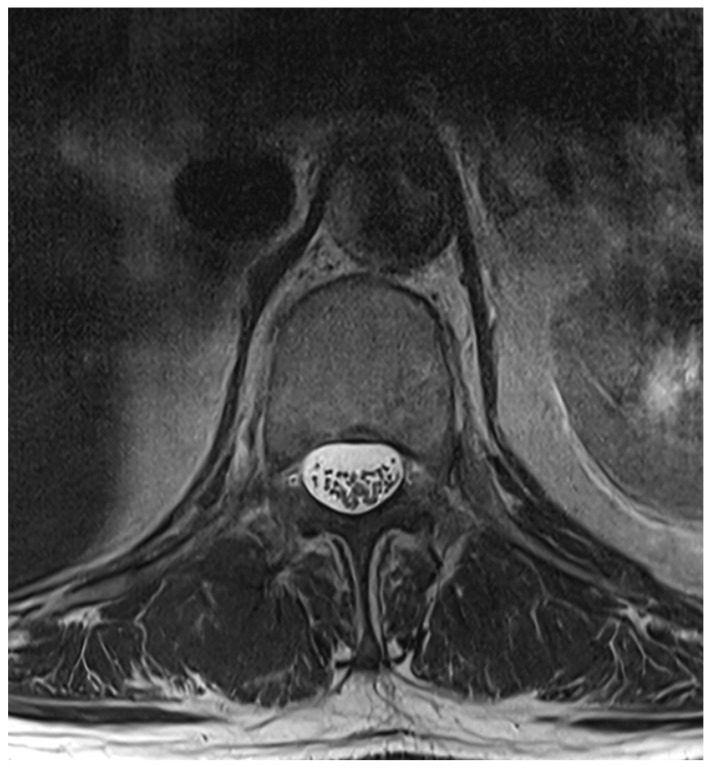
Axial T2 weighted MRI at L1, 6 weeks after blood patch. No epidural cerebrospinal fluid collections can be distinguished anymore.
